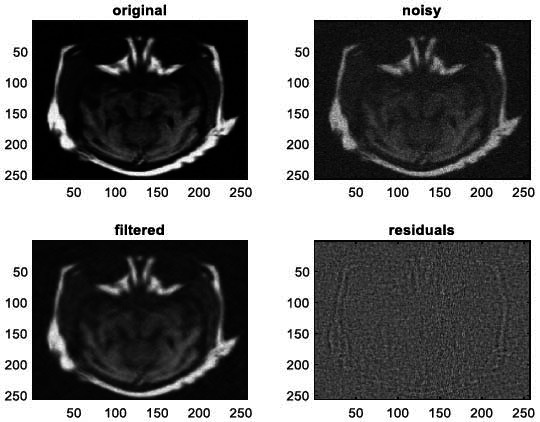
The eleventh example of MRI denoising by applying the proposed image denoising technique.
